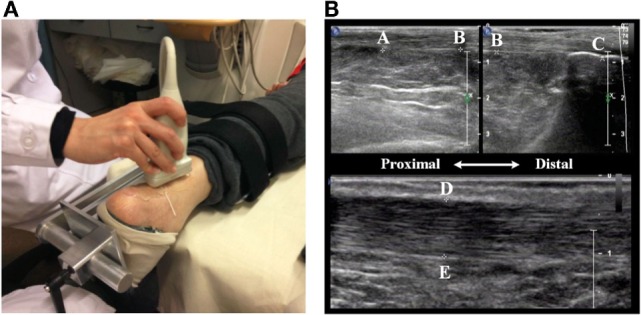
Ultrasonic measurement of AT. (A) The ankle is held at the natural position (90° between the leg and the foot) using a fixed apparatus. (B) The length of the AT is defined from the muscle–tendon junction (MTJ) (Point A) to its insertion into the calcaneus notch (Point C). The thickness of the AT is defined the distance between two points (D and E).
